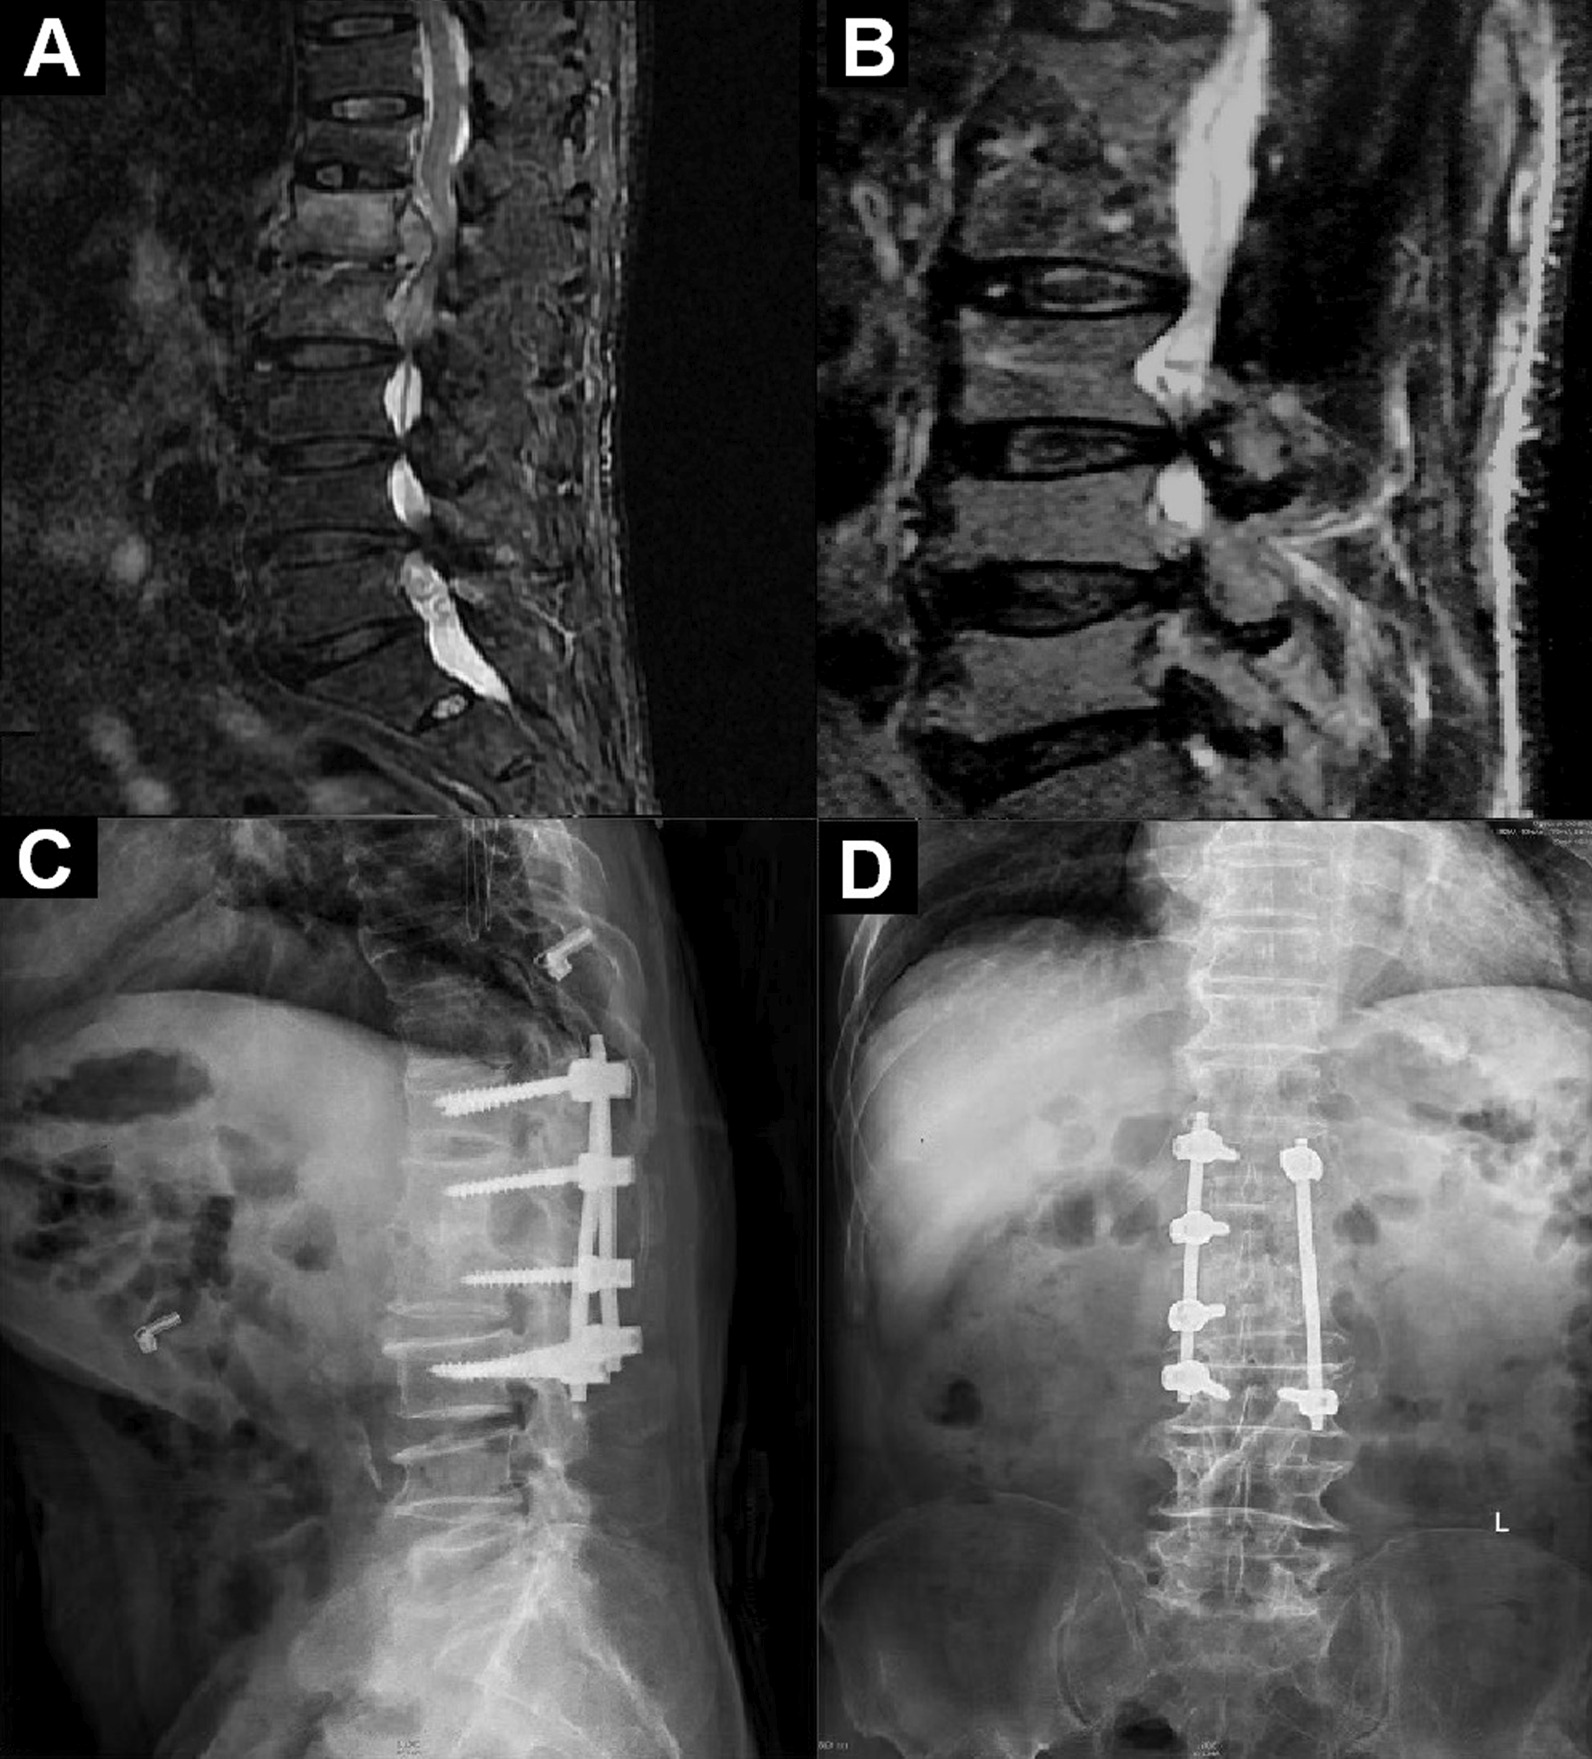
Magnetic resonance imaging (MRI) and radiograph of the patient before and after surgery (A T2-weighted imaging of the patient before surgery; B T2-weighted imaging of the patient 16 months after surgery; C Lateral radiograph of the spine 16 months after surgery; D Anteroposterior radiograph of the spine 16 months after surgery)
